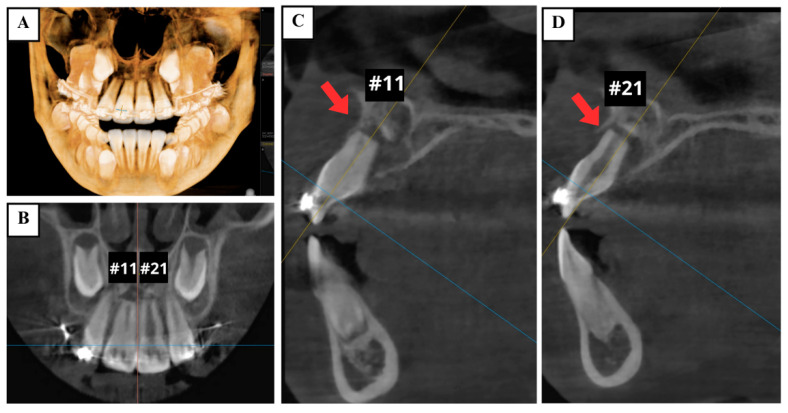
CBCT images at 14 months after orthodontic treatment showing satisfactory periodontal tissues around both the fractured fragments and the apical area (arrows). (A) 3D reconstruction. (B) Coronal view. Sagittal views of the (C) right maxillary central incisor (tooth #11) and (D) left maxillary central incisor (tooth #21).
